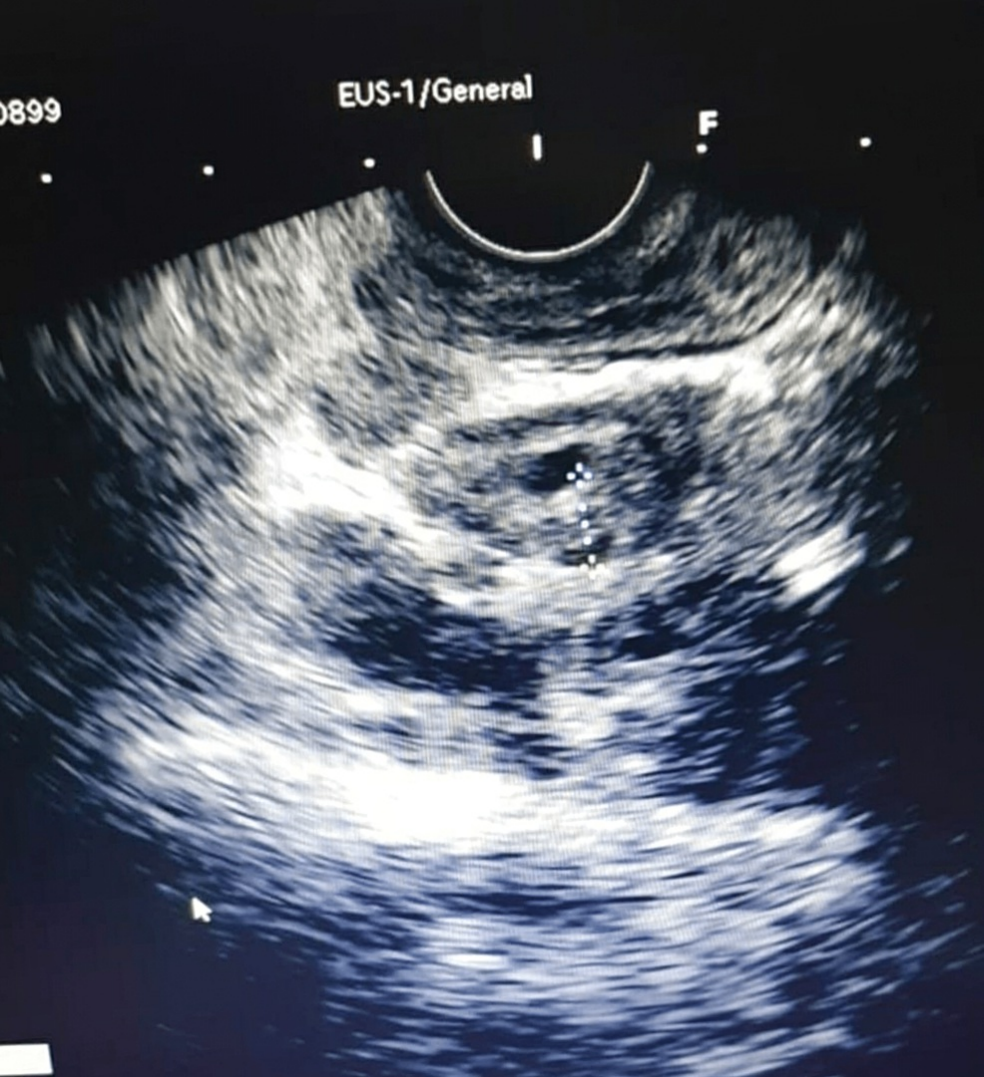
EUS showing CBD that is non-dilated (3 mm) but thick walled EUS, endoscopic ultrasonography; CBD, common bile duct
